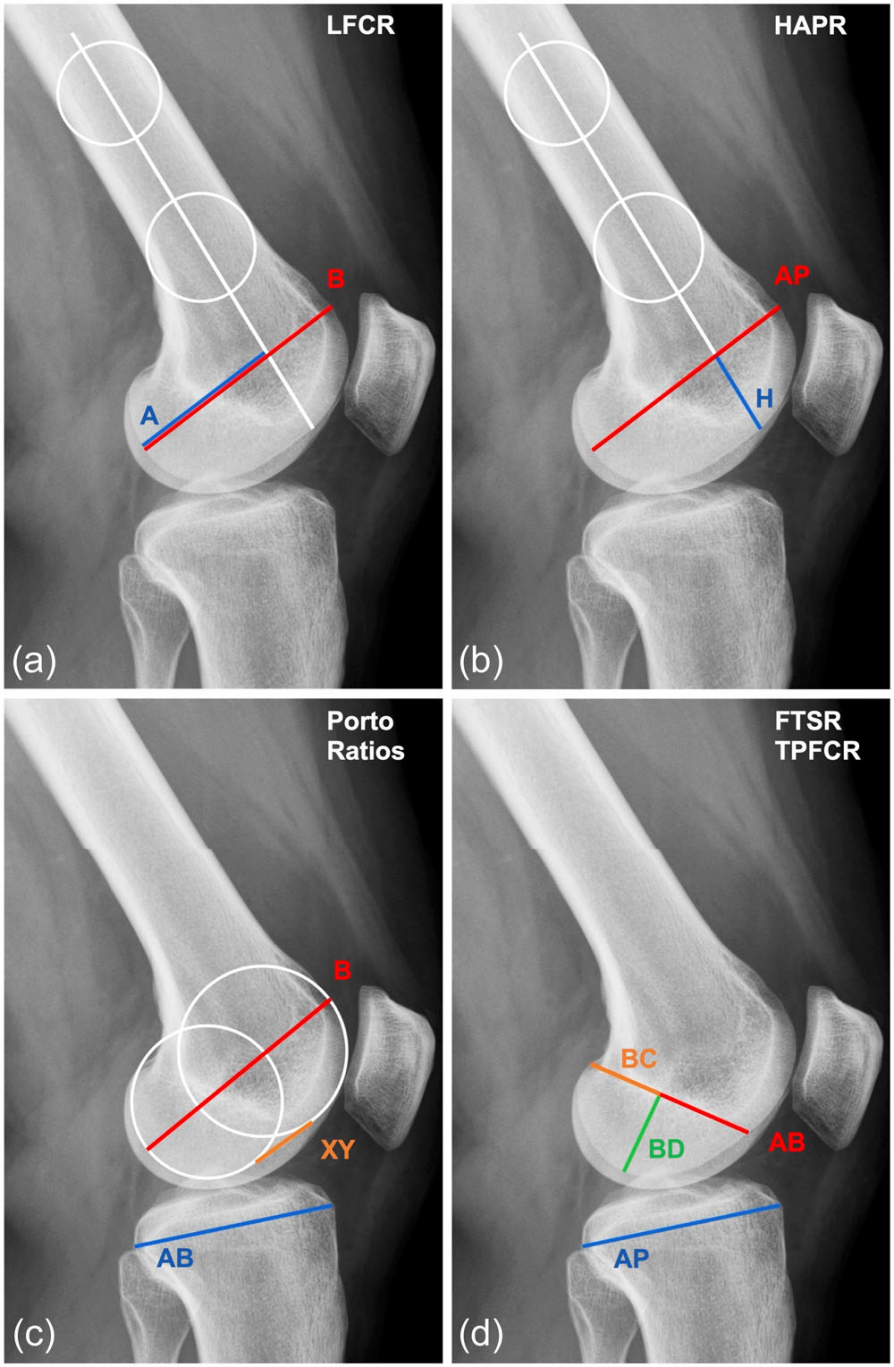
Different quantitative measurement methods of the lateral femoral condyle morphology on lateral radiographs. (a) Lateral femoral condyle ratio (LFCR); [22] (b) Height of lateral femoral condyle to anteroposterior diameter ratio (HAPR); [19] (c) Porto ratios (XY/AB, B/AB, B/XY); [26] (d) Femur tibia size ratio (FTSR) and tibia to posterior femoral condyle ratio (TPFCR) [3].
